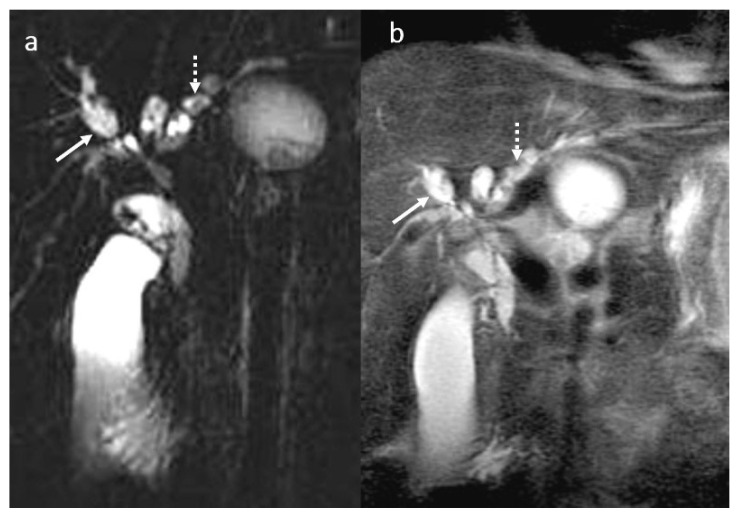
PSC in a male patient, 30 years old. MRI aspect before liver transplant: MRCP (a), T2 wi SSFSE short TE (b): multiple saccular and fusiform dilatation (arrow) in association with multiple short stenosis involving the intrahepatic and extrahepatic bile ducts with a moniliform appearance. Also note the presence of intrahepatic biliary lithiasis (dotted arrow).
